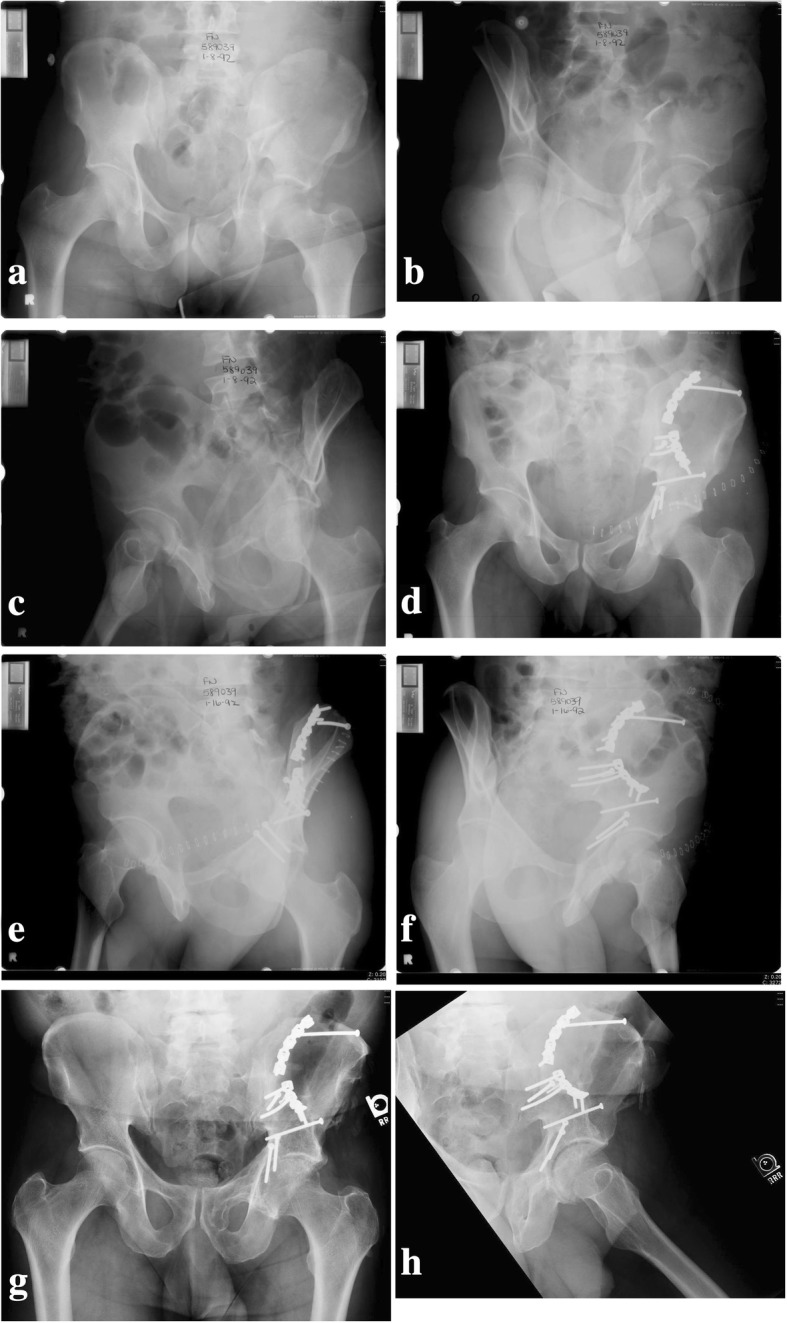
Pre-operative (a–c), immediate post-operative (d), and 21-year post-operative radiographs (e–h) of a patient after reduction and fixation of a both-column acetabular fracture. At 21 years, the left hip superior joint space is intact and patient has a good clinical outcome
